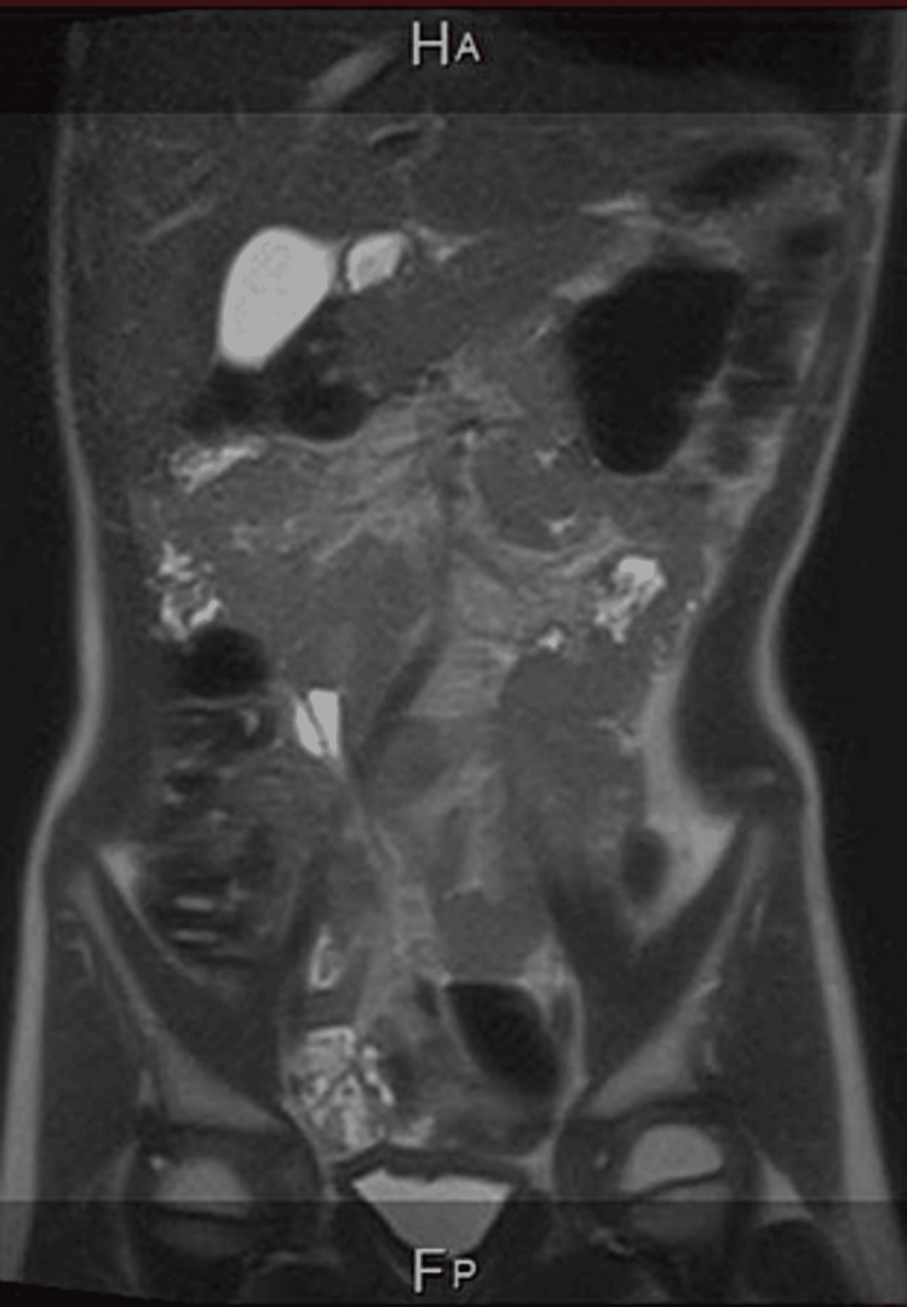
Coronal MRI of the abdomen shows complete resolution of the intra-abdominal lymphangiomas. MRI: Magnetic resonance imaging
